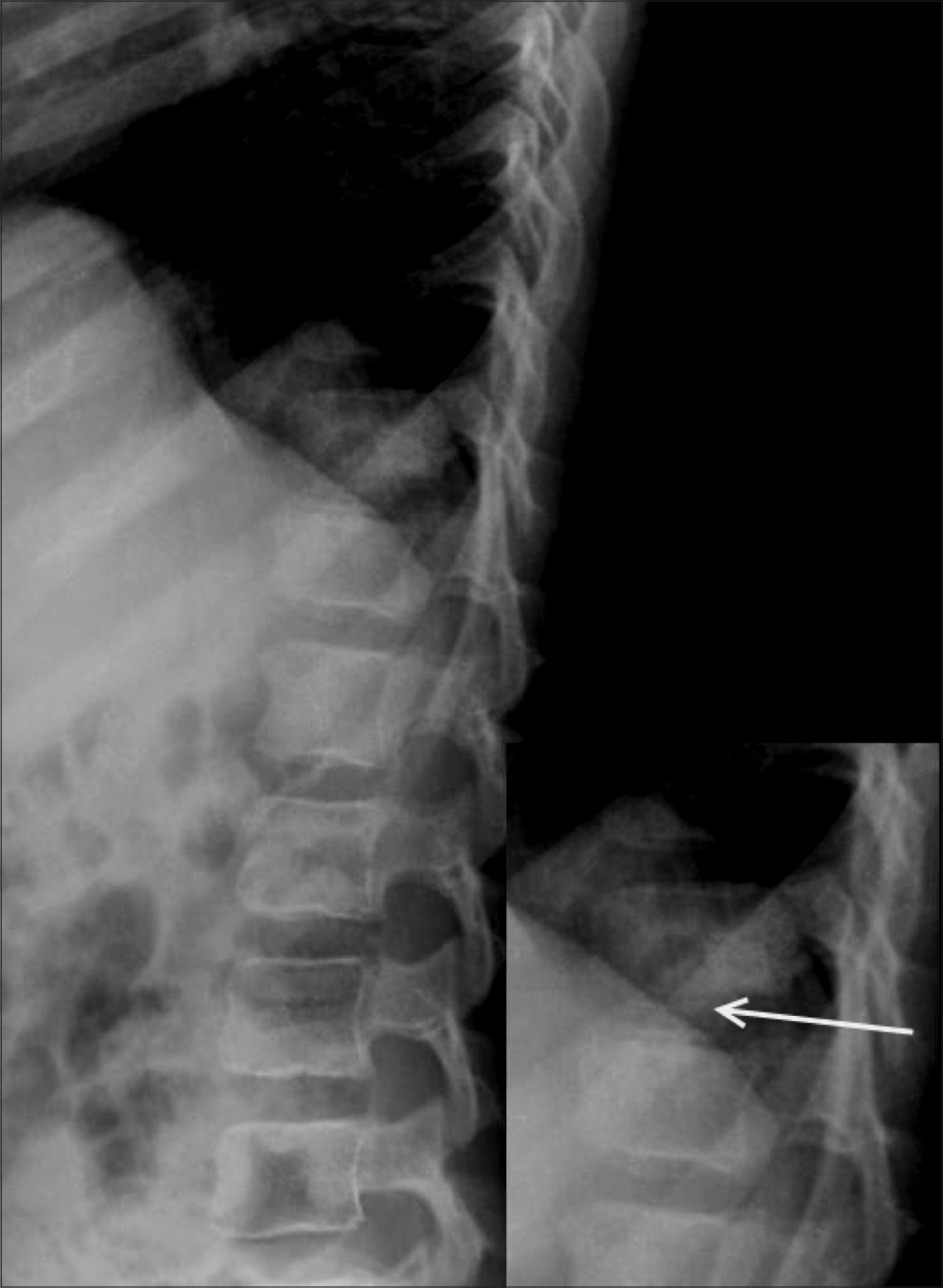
Lateral dorsolumbar spine radiograph obtained a day after the MRI scan shows calcification of the nucleus pulposus (arrow)
